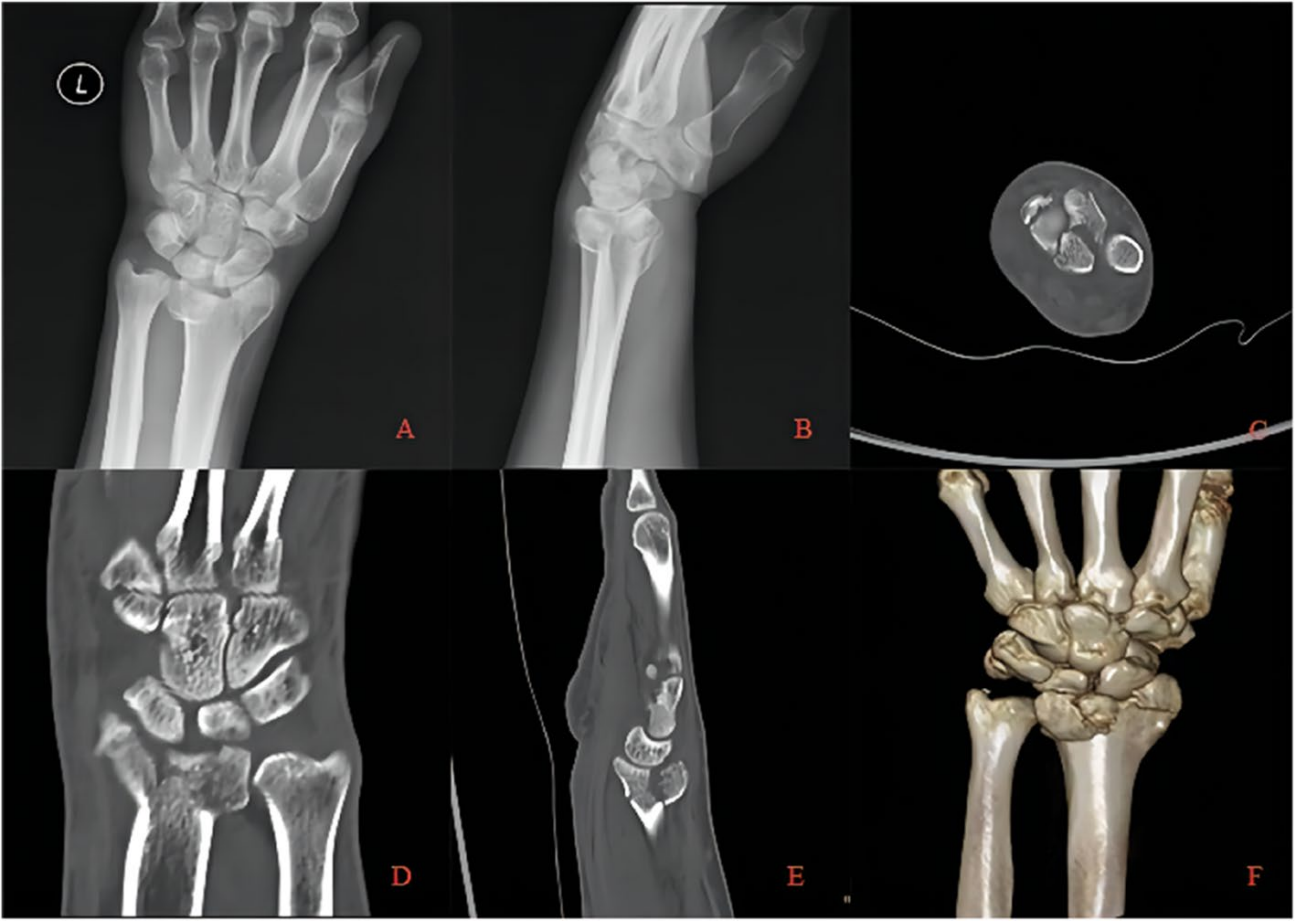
A 38-year-old patient with die-punch DRF. Radiography data showed a die-punch fracture of the left distal radius and a fracture of the articular surface of the intermediate column with obvious displacement. A AP radiography; B Lateral radiography; C Axial view of the articular surface of the radius. The fractures involved the articular surface of the intermediate column and lateral column; D Coronal plane, collapse of the lunate articular surface is obvious; E Sagittal plane, bone fragment shifted to the dorsal side; F Three-dimensional reconstruction of the CT image
